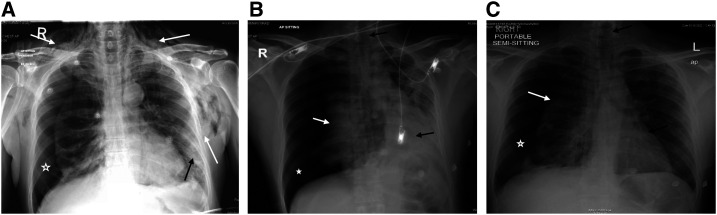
Chest X-ray (CXR) for three patients diagnosed with COVID-19 pneumonia. (A) Chest X-ray of case 1 showing bilateral neck and chest surgical subcutaneous emphysema (white arrows). Patchy infiltrates are noted in the left lower lung zone (black arrow). Right pneumothorax (star). (B) Chest X-ray of case 2 showing a large right-side tension pneumothorax (star), collapsed right lung (white arrow), deviated mediastinum (black arrows). (C) Chest X-ray of case 3 showing a right-sided pneumothorax (star), collapsed right lung (white arrow), and mild mediastinal shift toward the left side (black arrows).
